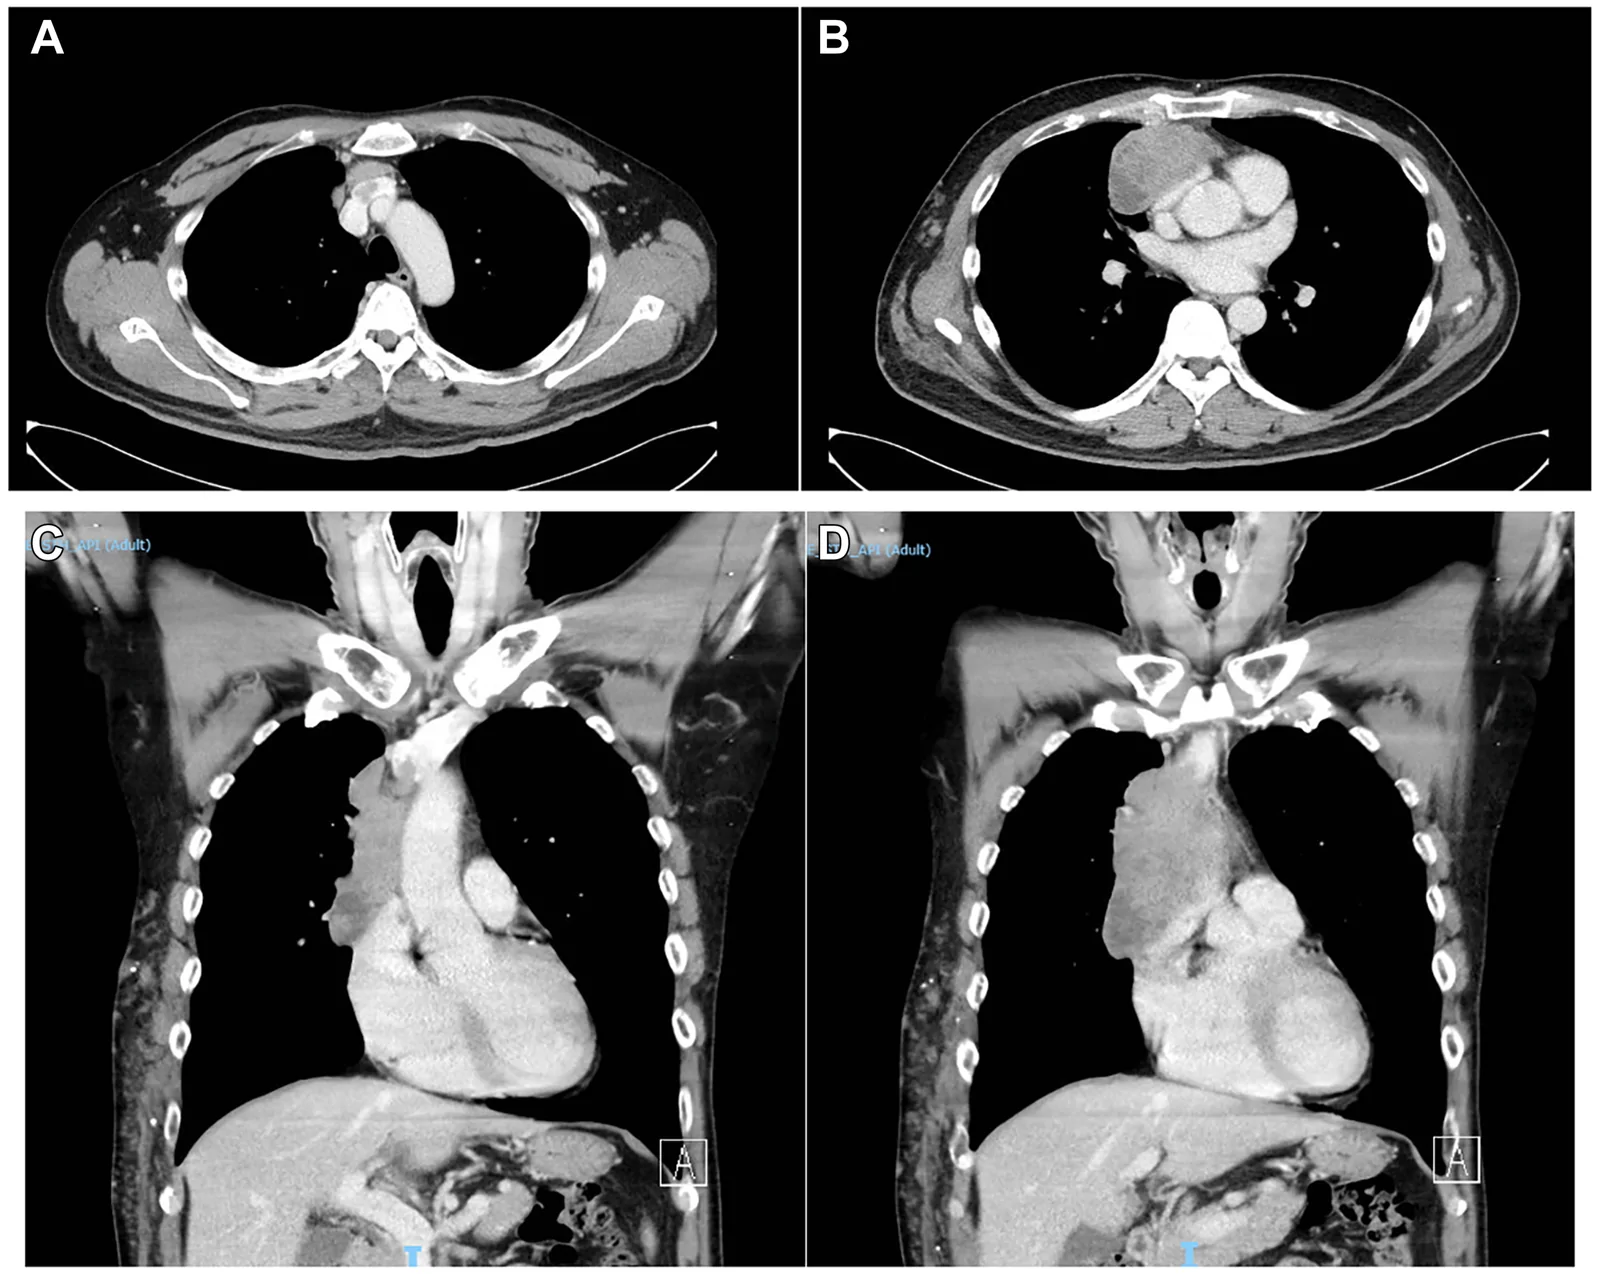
Computed tomography shows a right-sided anterior mediastinal mass invading superior vena cava and innominate veins. (A, B) Transverse views. (C, D) Coronal views.
